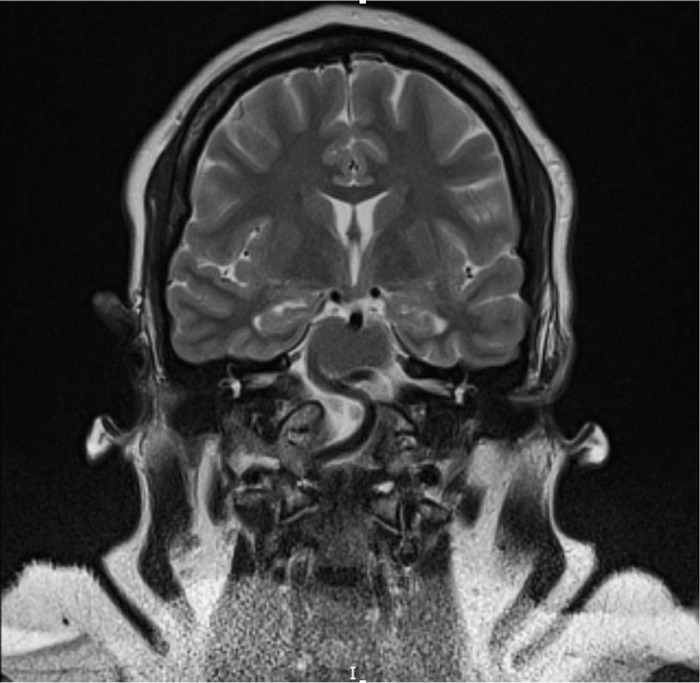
T2 weighted magnetic resonance imaging with contrast shows absent internal carotid artery flow voids through the cavernous segments (apparent absence of the bilateral internal carotid arteries). A tortuous and enlarged basilar artery passes adjacent to the origin of the sixth cranial nerve in the pons.
